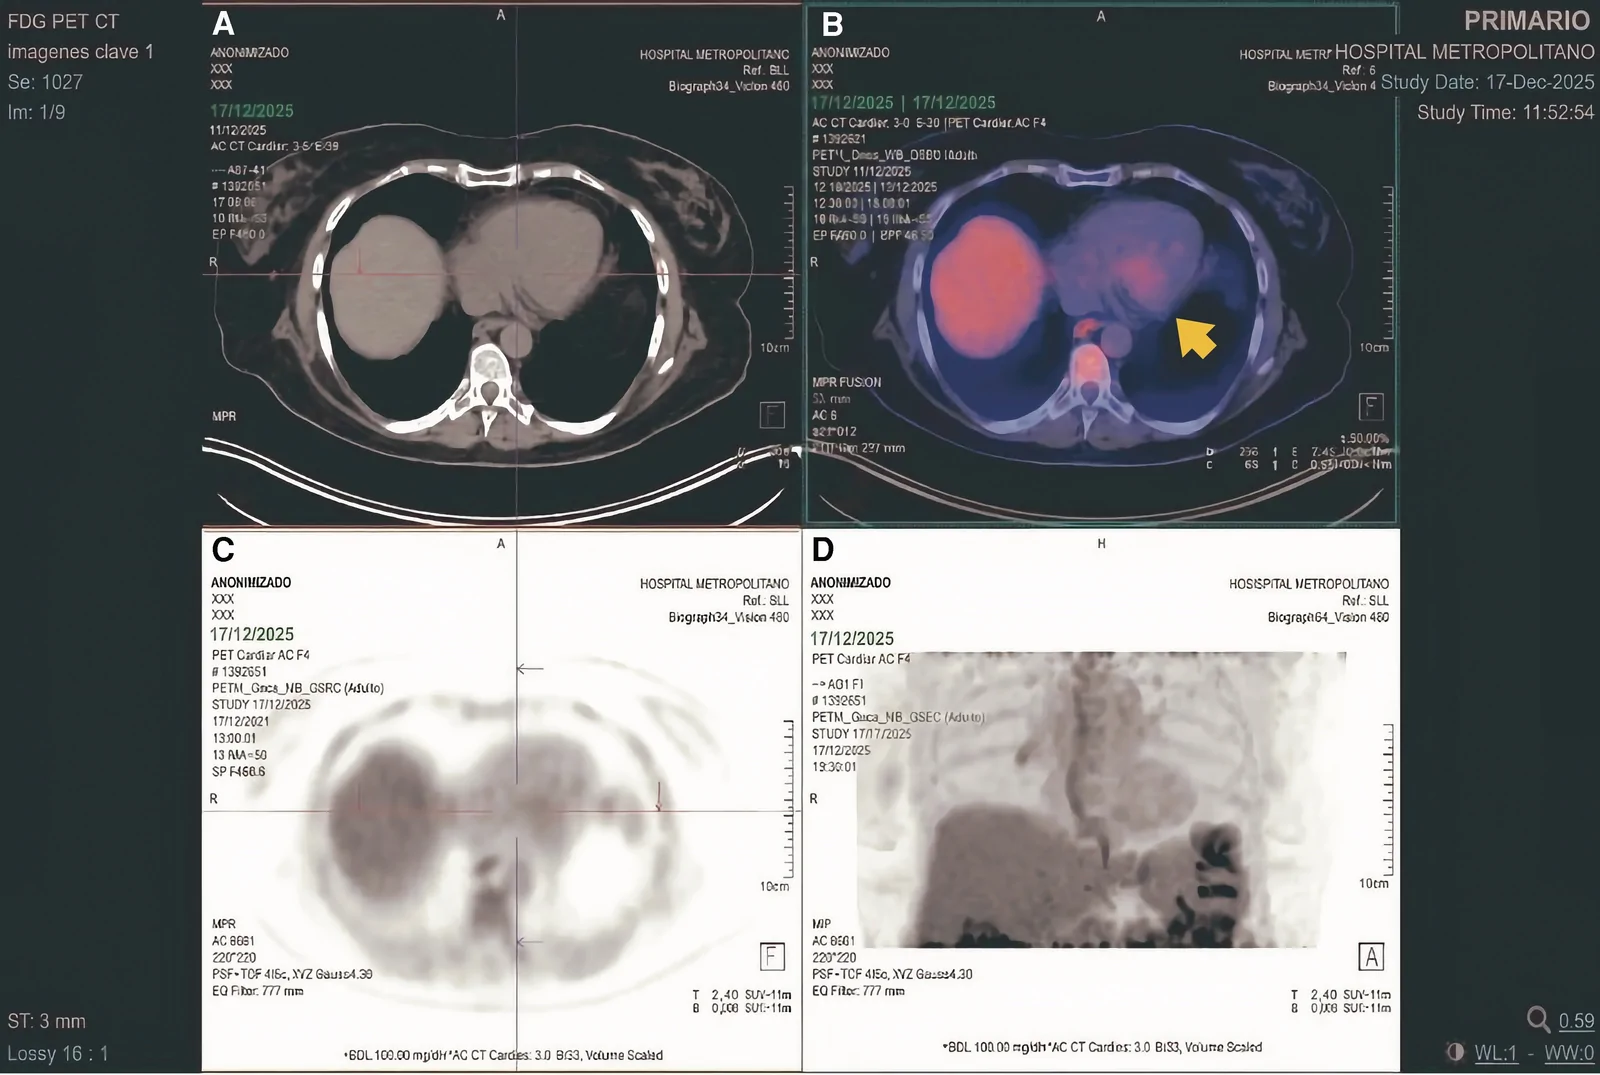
^18^F-fluorodeoxyglucose positron emission tomography demonstrating active myocardial inflammation. *(A)* Axial non-contrast computed tomography image showing preserved cardiac morphology without structural extracardiac abnormalities. *(B)* Fused positron emission tomography/computed tomography axial image demonstrating focal and multifocal myocardial ^18^F-fluorodeoxyglucose uptake predominantly involving the interventricular septum and left ventricular segments (arrow). *(C)* Axial positron emission tomography image confirming non-coronary patterned myocardial hypermetabolism on a well-suppressed background (arrows). *(D)* Whole-body maximum-intensity-projection image showing absence of extracardiac hypermetabolic lesions.
